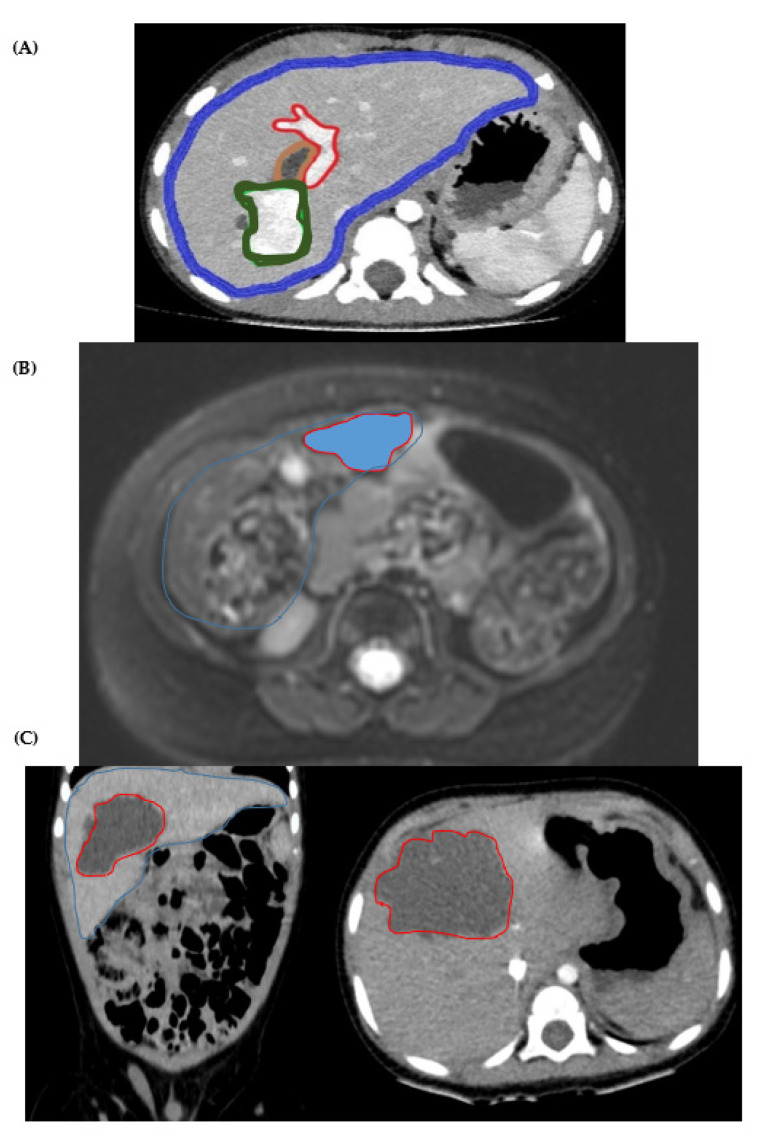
(A) CT image with the different anatomical parts highlighted: (1) Liver is outlined in blue; (2) Portal system is circled in red; (3) Dilated intrahepatic biliary tract (tumour) is outlined in brown; and (4) the rest of the tumour, which originates in the biliary tract, is circled in green. (Left) Coronal or frontal plane. (Right) Axial plane. (B) MRI of the case (Axial plane). The tumour is contoured in red. The liver is contoured in blue. (C) Left: MRI of the case (Frontal plane). Right: MRI of the case (Axial plane). The tumour is contoured in red. The liver is contoured in blue.
